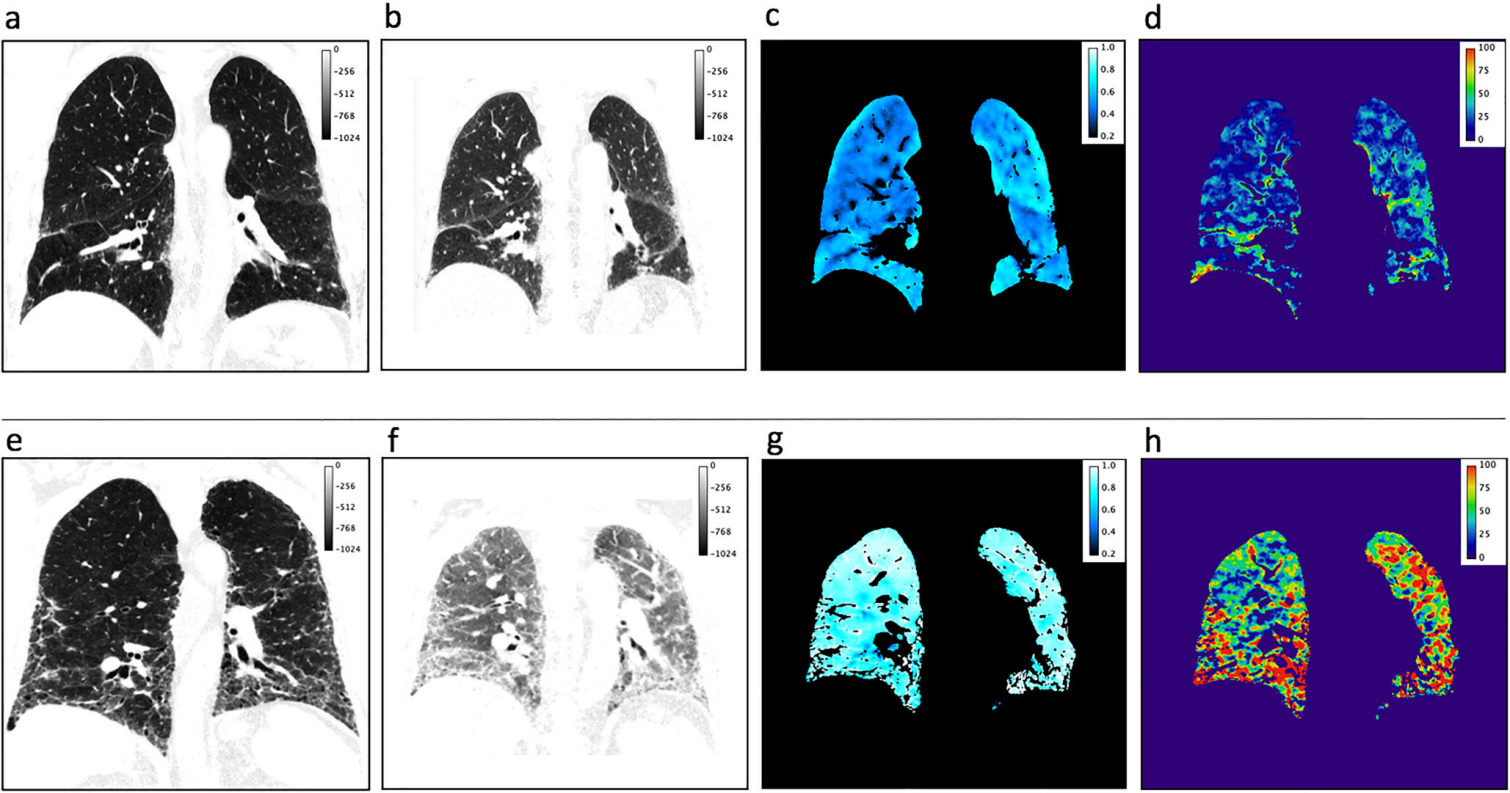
Baseline scans of a patient with stable disease (upper row, a–d) and a patient with progressive disease (lower row, e–h). The images are presented in the following order: CT in inspiration, CT in expiration, regional ventilation, and late enhancement. *Stable patient* mean regional ventilation 0.51; mean late enhancement 24%; baseline/follow-up values FVC% 73%/85%; lung volume 4269 mL/ 4549 mL; mean lung density - 812 HU/-827 HU. *Progressing patient* mean regional ventilation 0.76; mean late enhancement 52%; baseline/follow-up values FVC% 79%/57%; lung volume 3823 mL/ 3058 mL; mean lung density - 749 HU/− 677 HU. The patient in the upper row showed a low disease activity, as indicated in the regional ventilation and late enhancement images. FVC% and the mean lung volume and density values even improved with antifibrotic therapy. The patient in the lower row presented much higher ventilation and late enhancement values in the baseline scan, resulting in disease progression in the follow-up’s reference parameters
